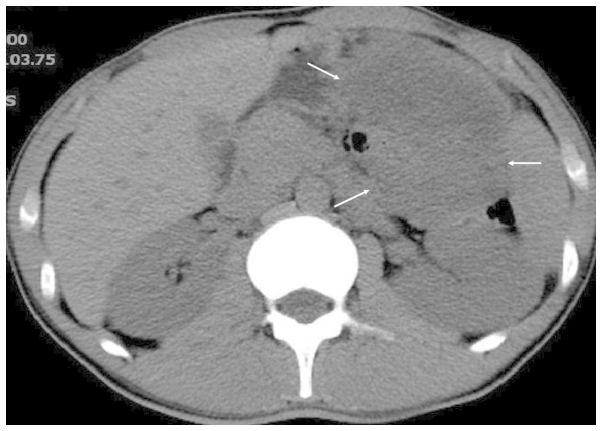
Transverse unenhanced computed tomography scan revealed an ill-defined and inhomogeneous mass, 12×10.6 cm in size, in the left upper quadrant (shown by the arrows). The mass occupied the left peritoneal cavity.
